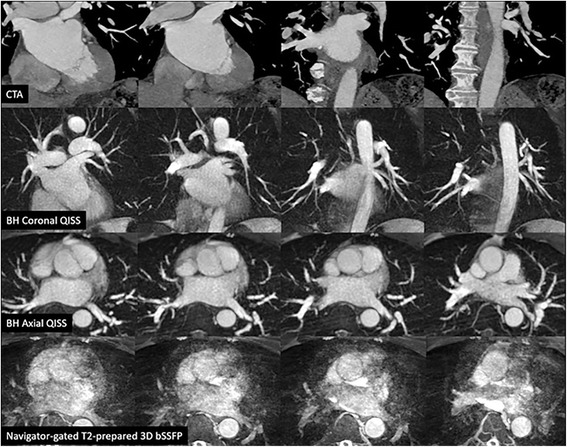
64-year-old male with poorly controlled atrial fibrillation and a rapid, variable RR interval (~480 ms) who underwent CTA and MRA prior to pulmonary vein isolation. All images are 12-mm thick maximum intensity projections. Single-shot radial QISS (*middle rows*) provided excellent depiction of the pulmonary arteries and veins in coronal and axial orientations despite the uncontrolled arrhythmia, whereas the navigator-gated 3D bSSFP images (*bottom row*) show severe artifacts
